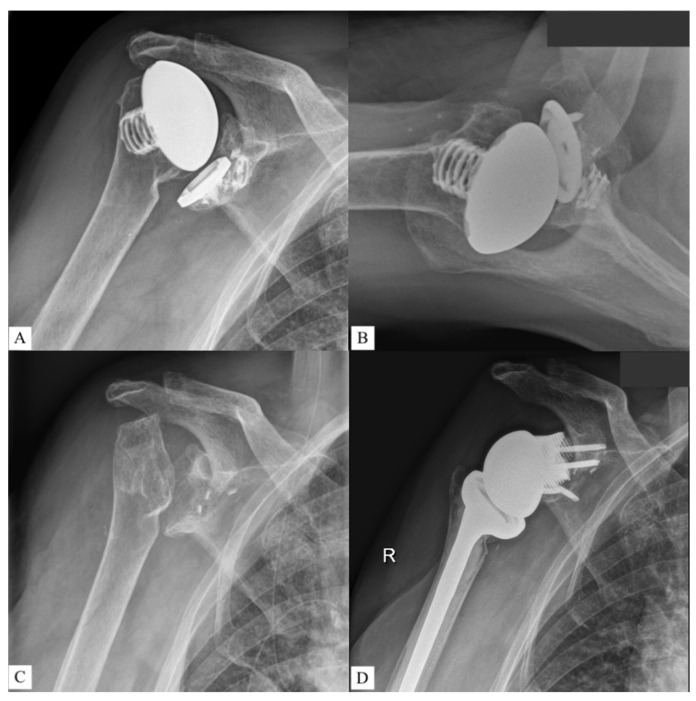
One case of revision reverse total shoulder arthroplasty severely damaged glenoid in the setting of implant loosening after anatomical TSA. Preoperative X-rays were performed in the (A) anteroposterior and (B) axial directions. (C) The surgical procedure consisted of two-time revision with explantation of the anatomical prosthesis and exclusion of infection via sonication and tissue samples. (D) Surgery using a custom-made glenoid component was performed after confirmation of aseptic loosening.
